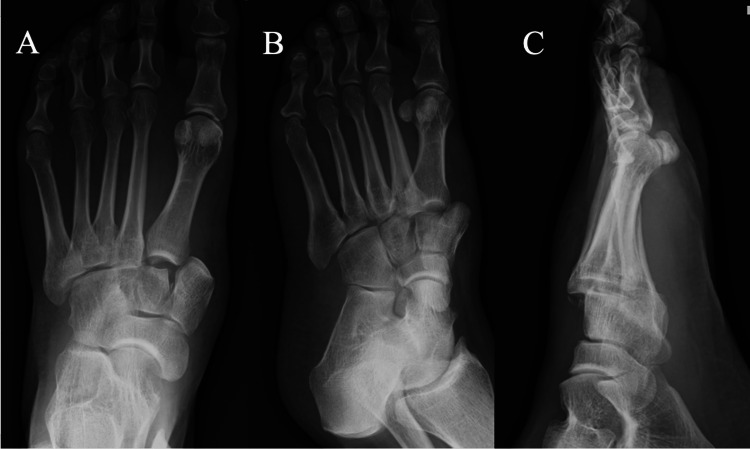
Preoperative radiographs of the left foot showing a Lisfranc fracture dislocation (A) Anteroposterior view. (B) Medial oblique view. (C) Lateral view. The images show the dislocation of the tarsometatarsal joint complex, widening between the medial and middle cuneiforms, and the key joints involved. These findings suggest marked midfoot instability and may raise suspicion of soft-tissue interposition in an irreducible Lisfranc injury.
